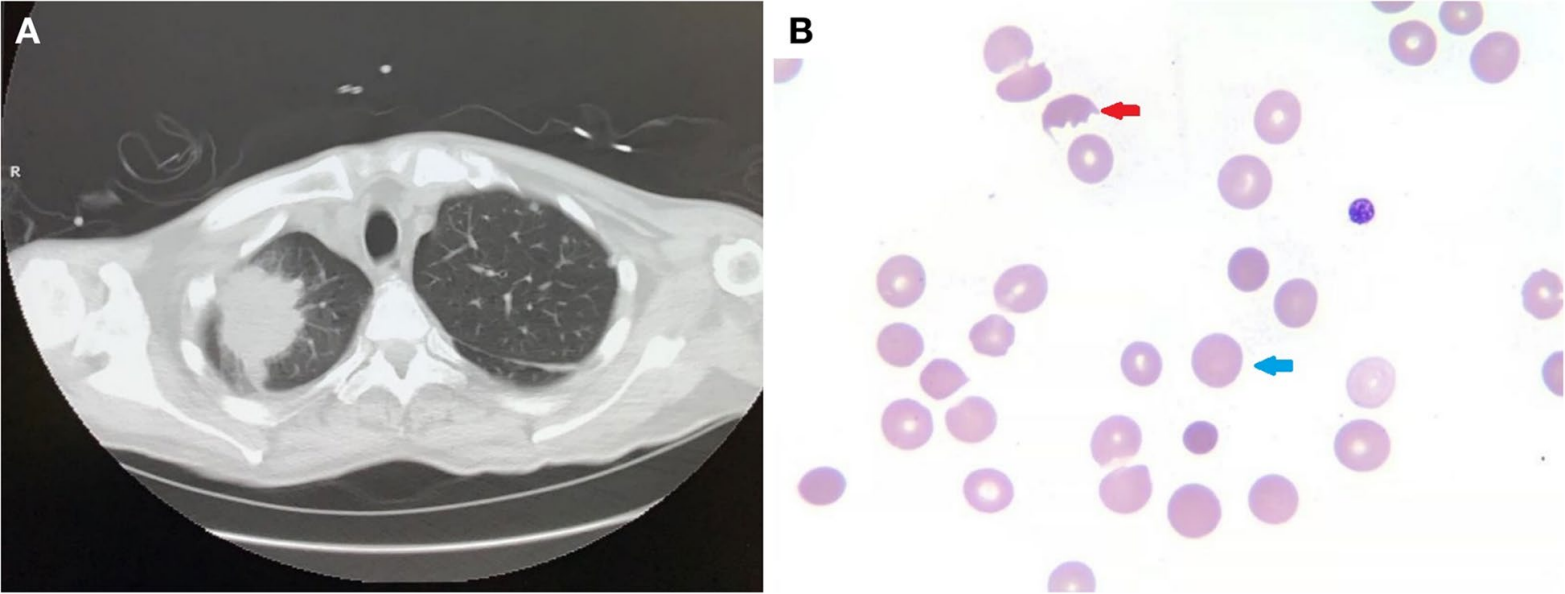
A the CT scan showed the tumor at the right upper lobe of the right lung, B The schistocytes (red arrow) and spherocytes (blue arrow) could be seen under this field of vision in peripheral blood smear
